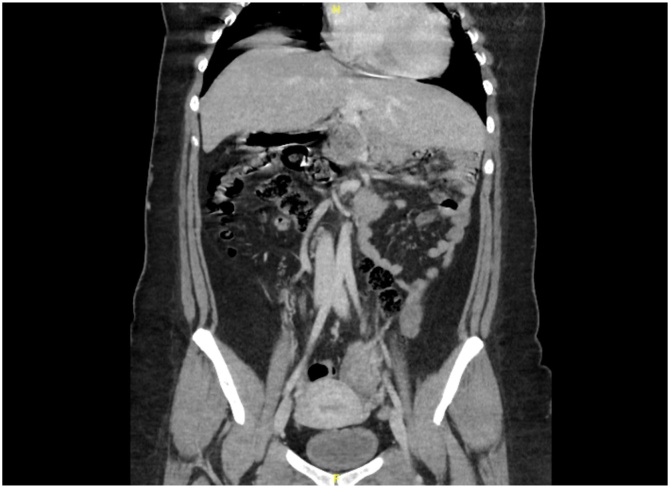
This is a coronal view of the CT scan showing the midline liver.
